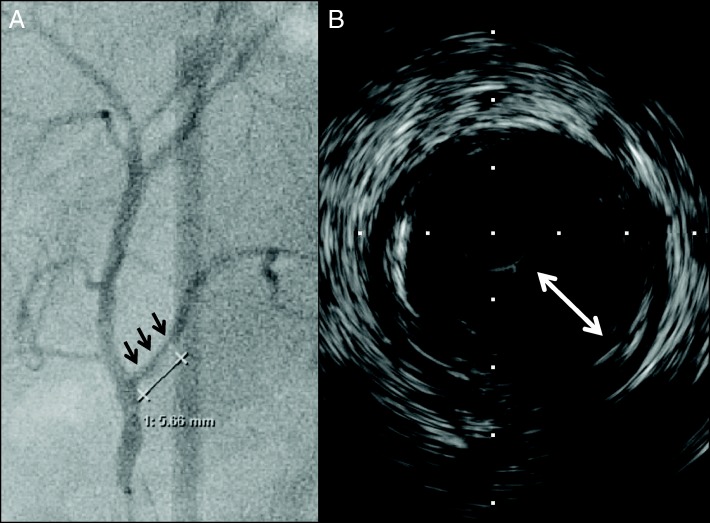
Digital subtraction angiography (a) shows stricture of the right posterior hepatic artery (arrows), and thick plaques (double-head arrow) in the arterial intima were observed by intravascular ultrasonography (b)
